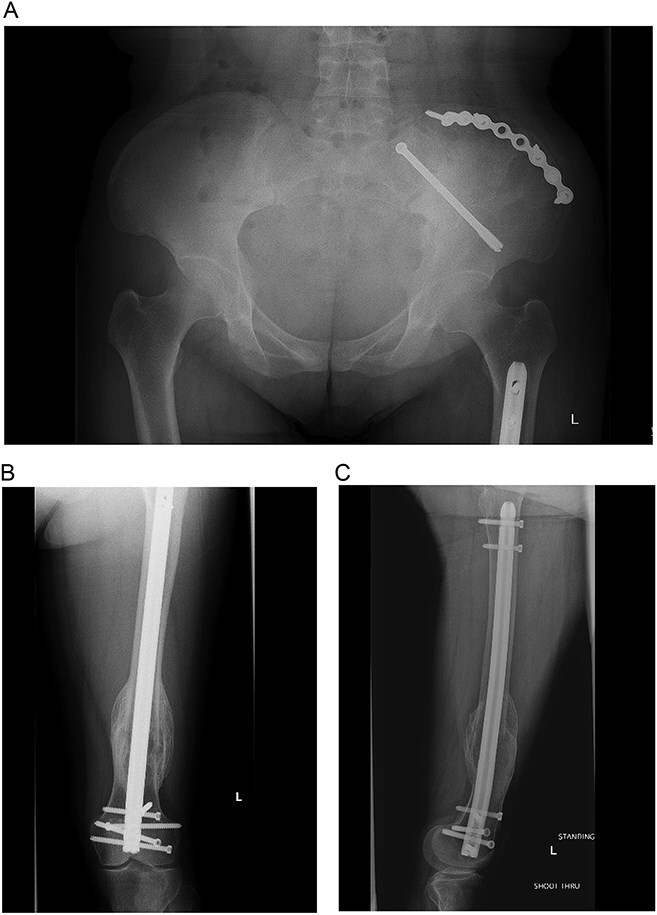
(A–C) Final radiographs showing complete healing >1 year postoperatively.
